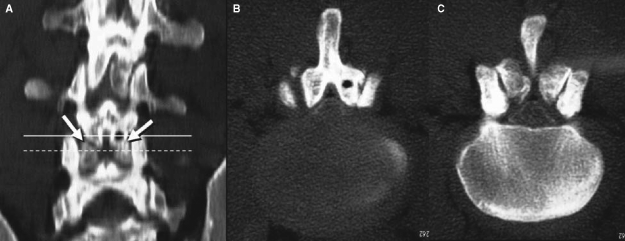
Contrast-enhanced spinal CT scan. A: Frontal plane reconstruction clearly demonstrated spondylolysis of the bilateral inferior articular processes of the L4 vertebra (arrows). B: Horizontal sections at L4/5 disc revealed sagittalization of the L4/5 facet, and deviation of the inferior articular processes of L4 to the right and anterior direction which compressed the dural sac. C: Horizontal sections at the superior margin of the L5 vertebra revealed bone fragments separated from the inferior articular processes of L4.
